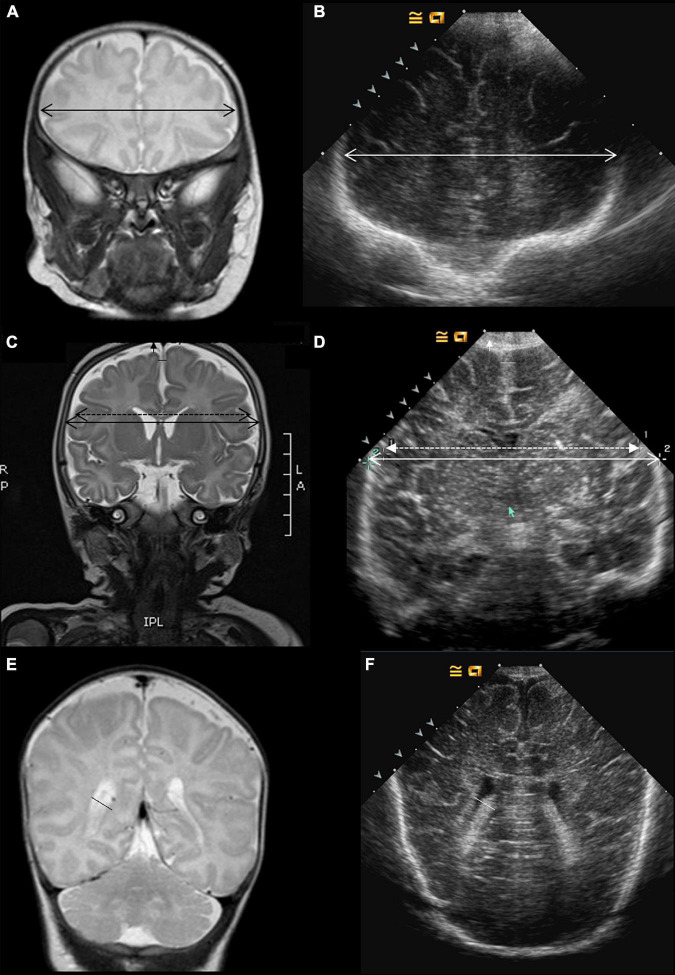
Imaging metrics protocol. MRI and ultrasound coronal views. The following metrics were compared on coronal, T2-weighted MRI sequences (A,C,E) and ultrasound scans (B,D,F): bi-frontal diameter (A,B), and lateral ventricle diameter (E,F). On panels (C,D), the bone parietal diameter is shown in plain double head arrow and the brain parietal diameter in the discontinued double head arrow. Measurements of extra-cerebral spaces include the subarachnoid (vertical arrowhead) and the inter-hemispheric spaces (horizontal space).
